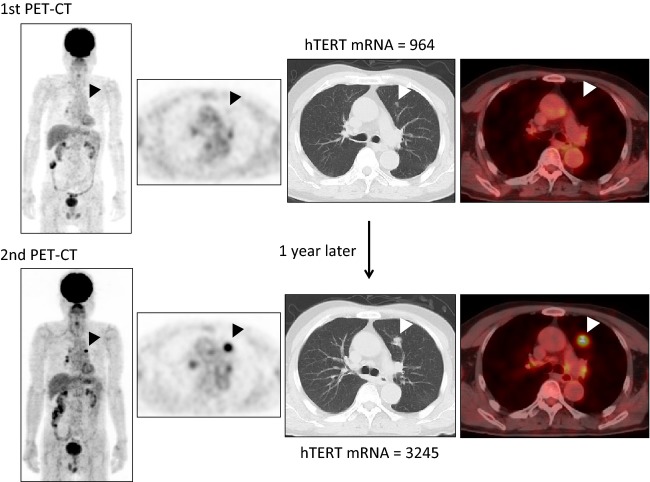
A representative case of a patient with lung cancer. The diagnosis was confirmed by needle aspiration cytology. The top and bottom columns include four images (PET-CT, axial view of PET [MIP: image], axial view of CT, and fusion image [selected transaxial fused PET/CT slice] from the right to left); the bottom images were acquired a year after the top images to enable a more precise diagnosis. The fusion image was used for enhancing FDG accumulation on selected slice. The first PET-CT study did not diagnose the findings (shown by an arrowhead) as indicative of malignancy yet. The hTERT mRNA increased from 964 to 3245 copies in the 1-year interval between imaging studies, with both values indicating positivity. The bottom images demonstrate the increased size of a lung tumor over time, suggesting the potential use of hTERT mRNA quantification as a complementary diagnostic technique. PET, positron emission tomography; CT, computed tomography; MIP, maximum intensity projection; FDG, fluorodeoxyglucose; hTERT, human telomerase reverse transcriptase.
